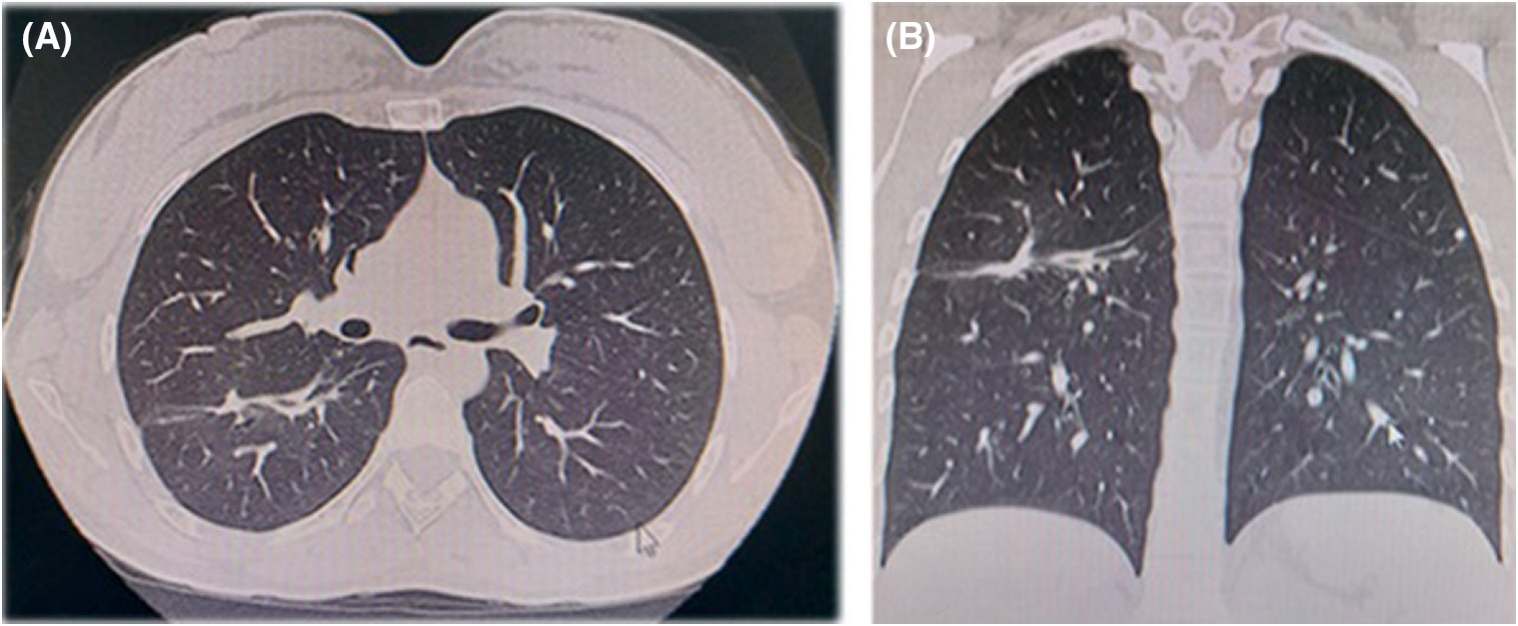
(A) Axial computed tomography view, showing thickening of the major fissure of the right lung associated with a small hyperdense image of irregular subpleural shape adjacent to the major fissure of the lower lobe, as residual changes in relation to pathological and surgical history. (B) Coronal computed tomography view.
